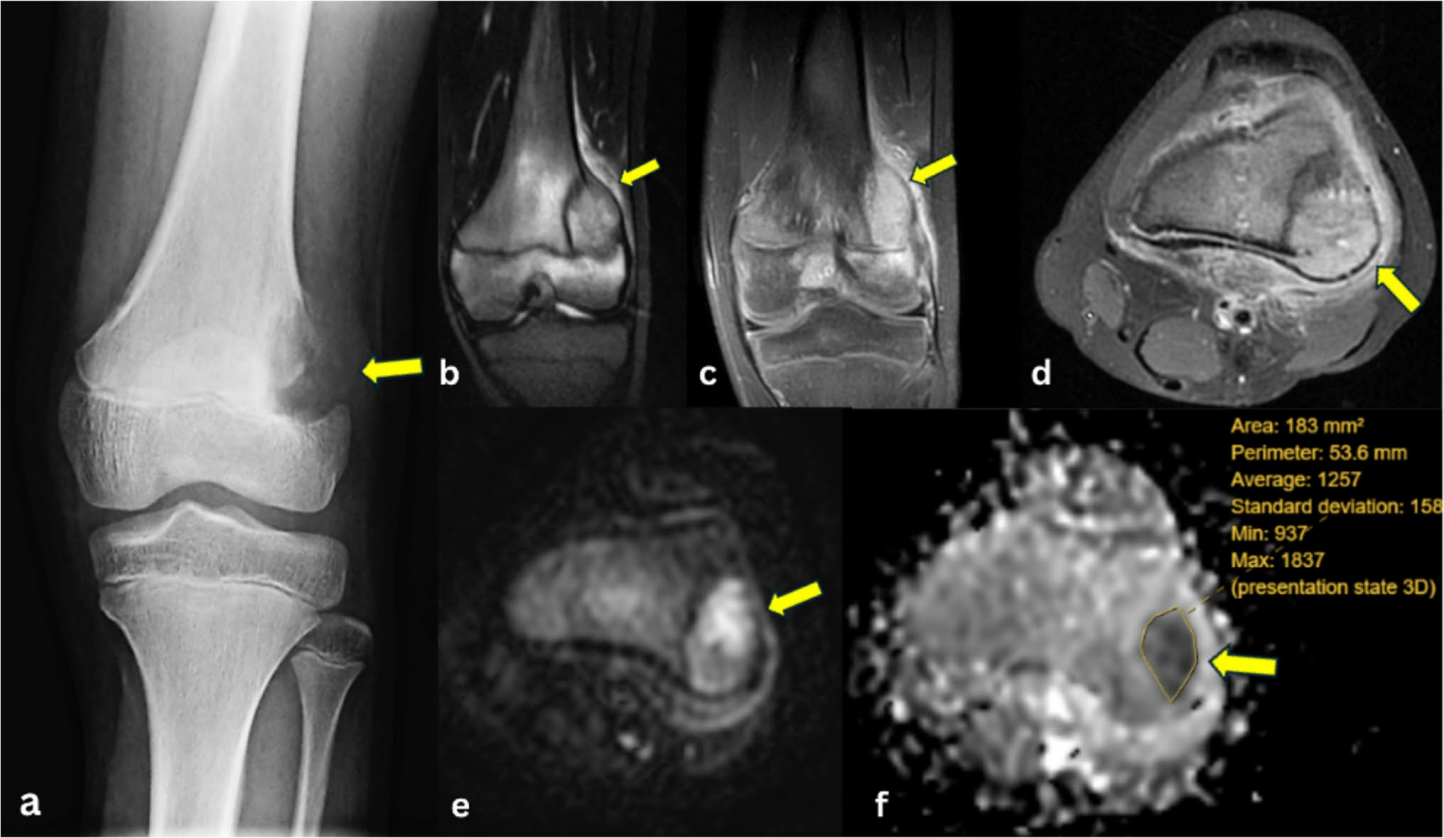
Chondroblastic osteosarcoma of the femur in a 13-year-old female. a AP radiograph of the left knee shows an aggressive appearing osteolytic lesion (arrow) arising from the lateral aspect of the distal femoral metaphysis, with destruction of the lateral cortex. b Coronal short tau inversion recovery (STIR) MR image demonstrates a hyperintense lesion (arrow) in the distal femur metaphysis which extends across the growth plate into the epiphysis, and which disrupts the lateral cortex. c Coronal post-contrast fat-suppressed T1-weighted image shows that the lesion enhances with contrast (arrow). d Axial post-contrast fat suppressed T1-weighted image demonstrates inhomogeneous enhancement of the lesion, (arrow). e Axial DW-MRI reveals a hyperintense lesion (arrow) in the left distal femur. f Corresponding ADC map shows a hypointense signal (arrow), indicating restricted diffusion, with ADCmean of 1.25 × 10^−3^ mm^2^/s; ADCmin of 0.93 × 10^−3^ mm^2^/s, and ADCmax of 1.83 × 10^−3^ mm^2^/s
